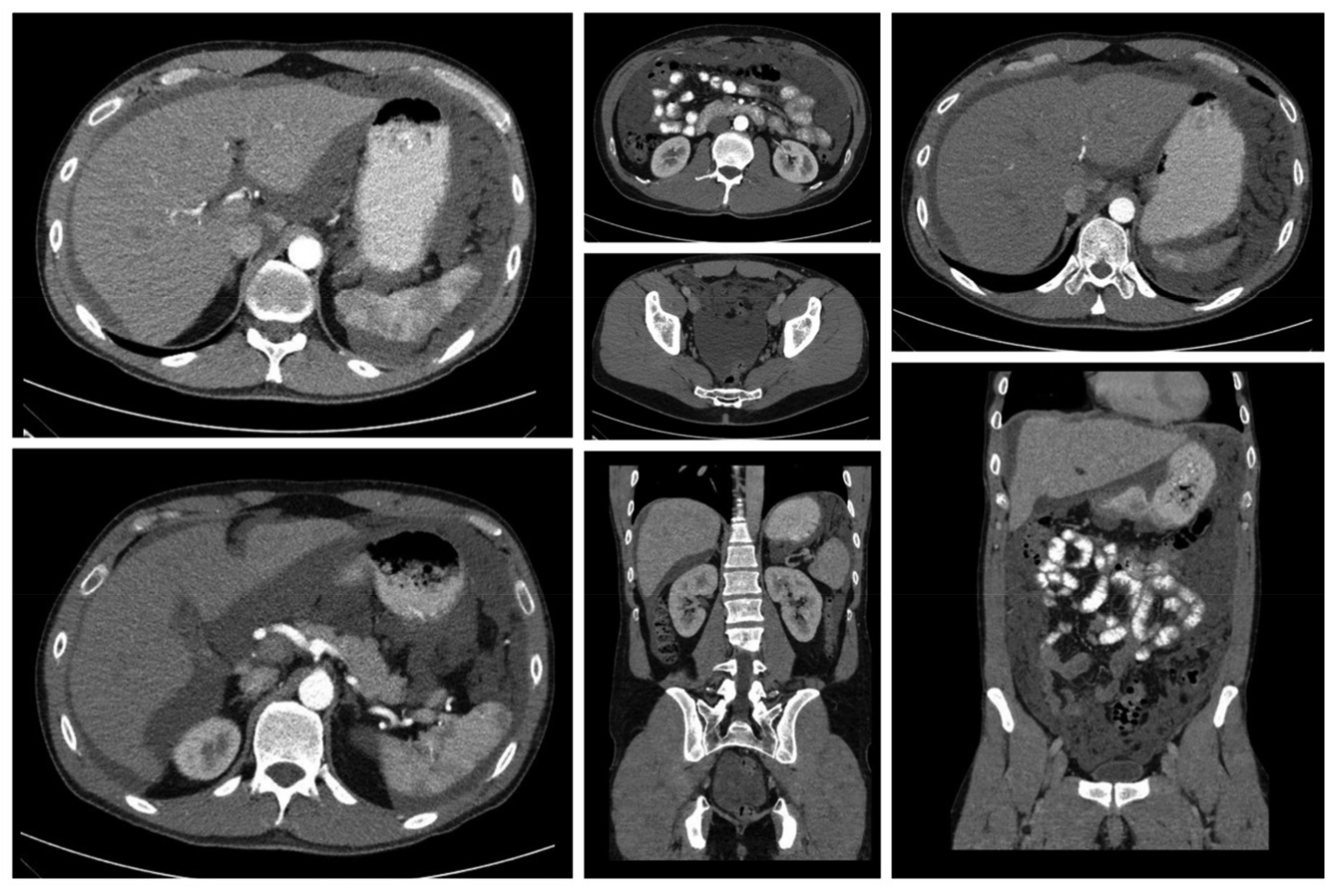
CT images of a patient with extensive PMP tumor burden.
